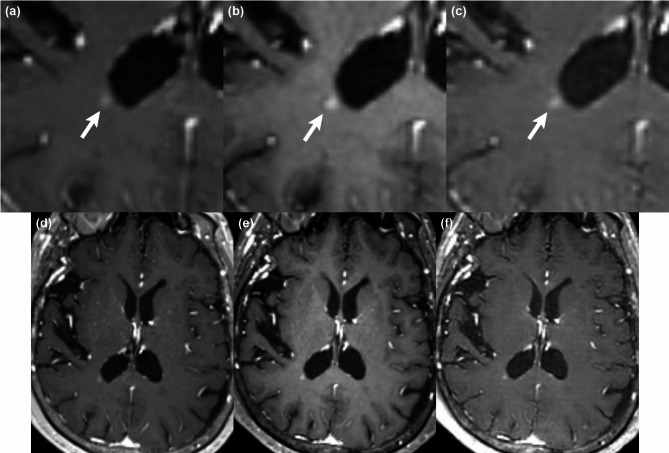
An 83-year-old woman with lung cancer. As compared with the baseline image (a), a small contrast-enhancing lesion (arrow) at the right temporal lobe appears slightly more discrete and larger due to a higher degree of lesion-to-white matter contrast on the original FU image (b). On the harmonized FU image (c), the lesion appears similar to that of the baseline image in terms of the border, size, and contrast enhancement. Note that the gray matter-to-white matter contrast is higher on the original FU image (e) than on the baseline (d) or harmonized FU image (f). The baseline and FU images were scanned with Magnetom Skyra (Siemens Healthineers) and Ingenia 3.0T CX (Philips Healthcare) MRI scanners, respectively.
